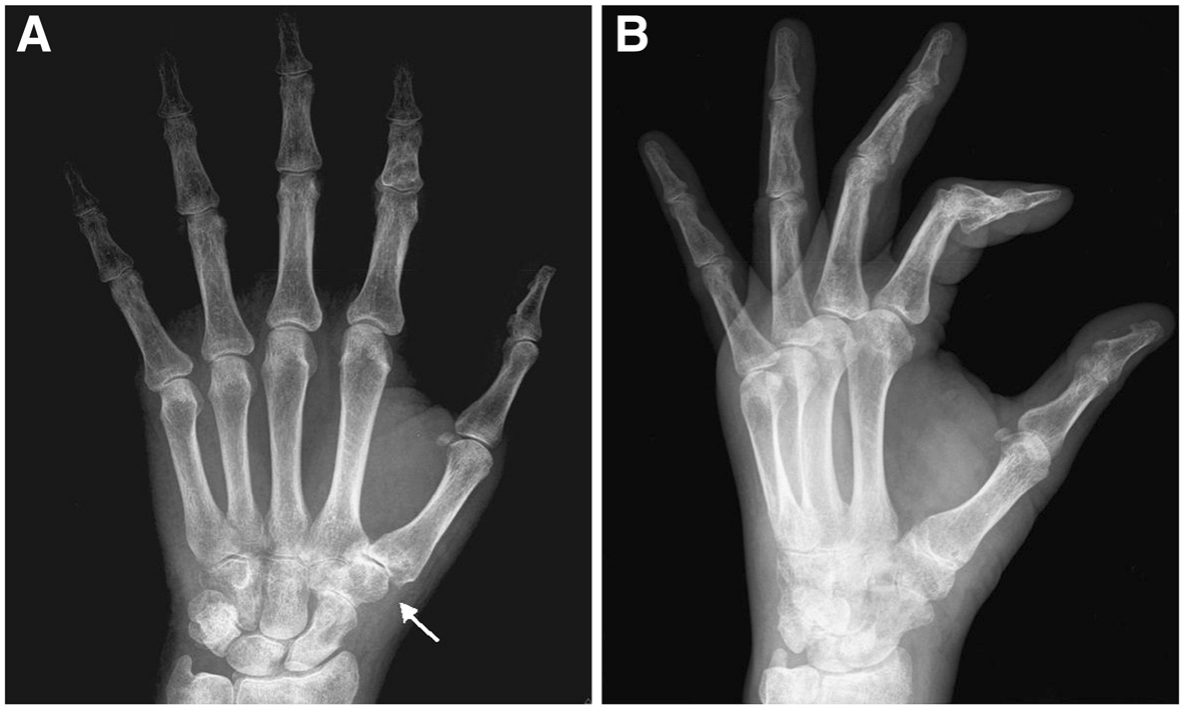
Plain radiograph of the hand performed after the second clinical examination. (A) Anteroposterior view. (B) Lateral view. Space narrowing of the trapeziometacarpal and trapezionavicular joints (*arrow in *[A]). Diffuse osteoporotic picture of the carpal and the phalangeal bones, to be interpreted as disuse osteoporosis.
